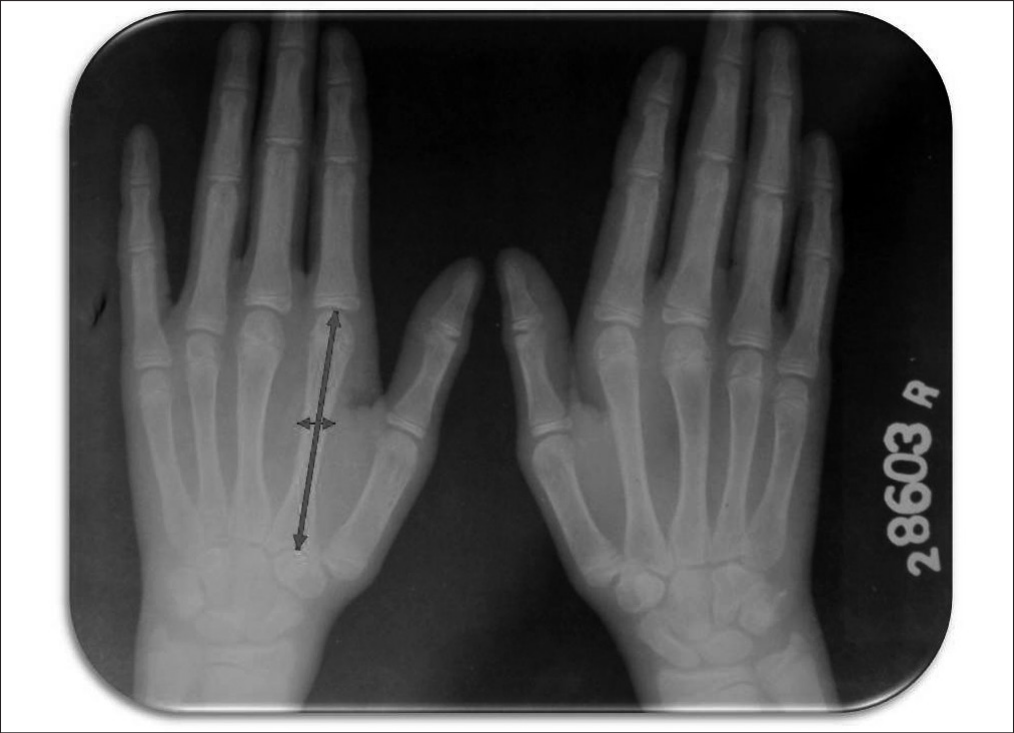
X-ray of hands. Note elongation of the metacarpals
